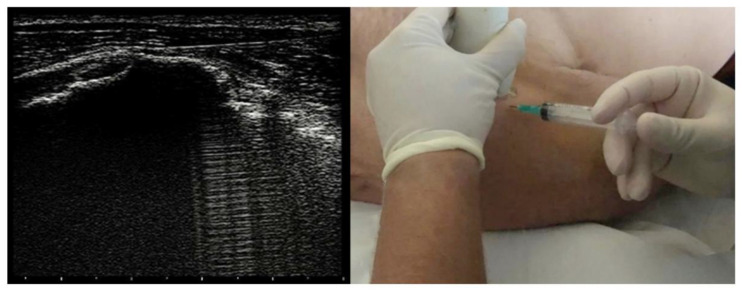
Ultrasound-guided right subacromial bursa injection via anterior approach.
